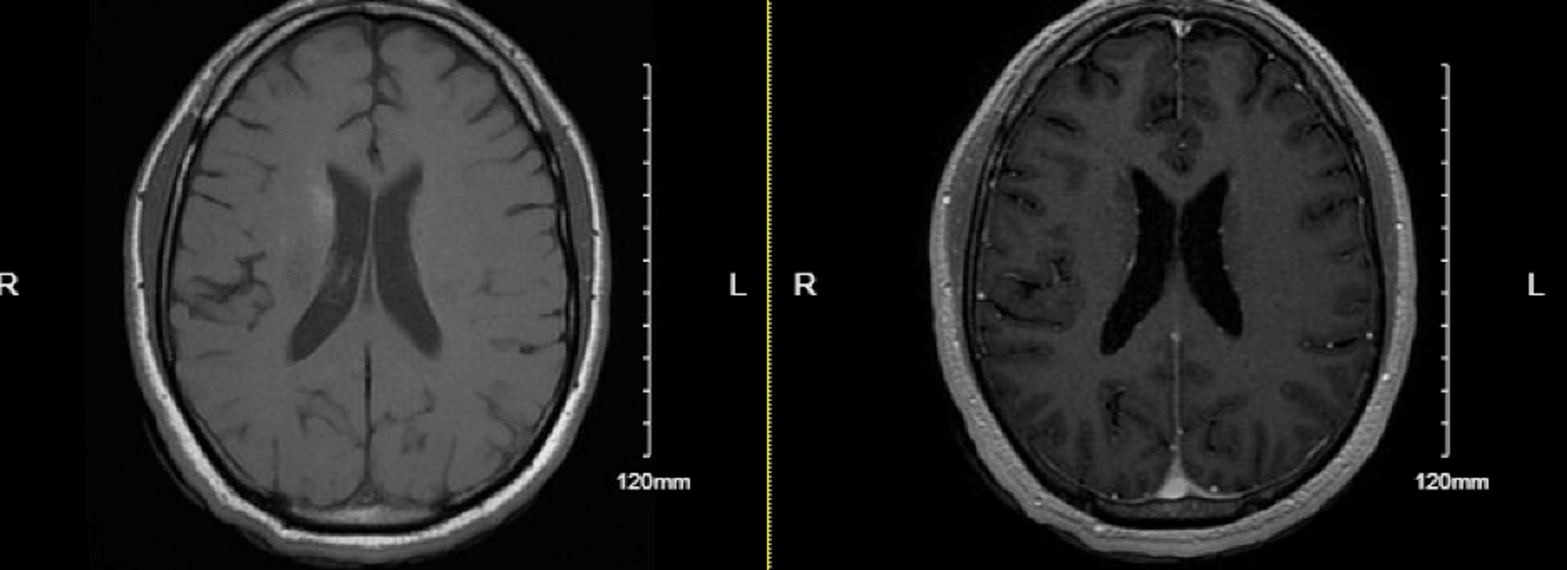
One-month post treatment MRI non-contrast Radiology impression includes interval improvement in areas of T2 prolongation, which have enhancement in the parenchyma and leptomeninges within the bilateral cerebellar hemispheres and right basal nuclei in comparison with initial MRI of the brain. Minimal residual enhancement remains. No midline shift or uncal herniation are present. No new areas of abnormal enhancement within the cerebral or cerebellar parenchyma are present.
